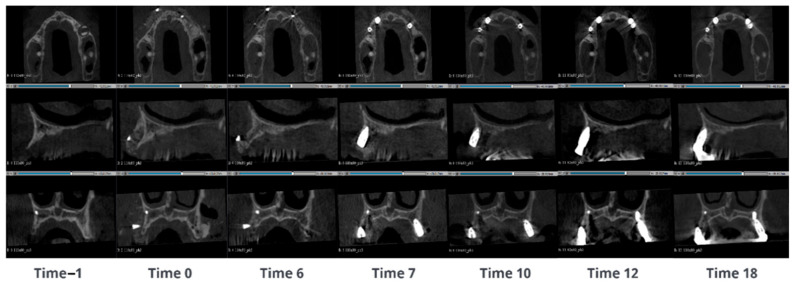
CBCT images of a 58-year-old woman with devitalization of multiple teeth and bone resorption in the maxillary. SmartBone^®^ On-Demand plates were inserted on both sides of the front maxillary. The images show the progression of bone healing over time, from pre-surgery (Time −1) to 18 months post-surgery (Time 18).
